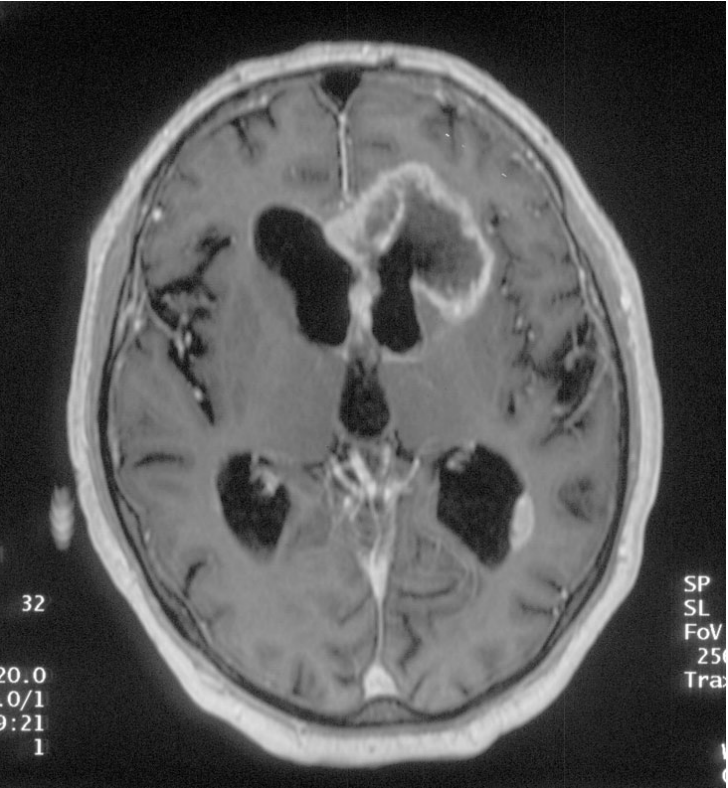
Magnetic resonance imaging of the brain revealed a left frontal, invasive, multilocular, infiltrating process.
